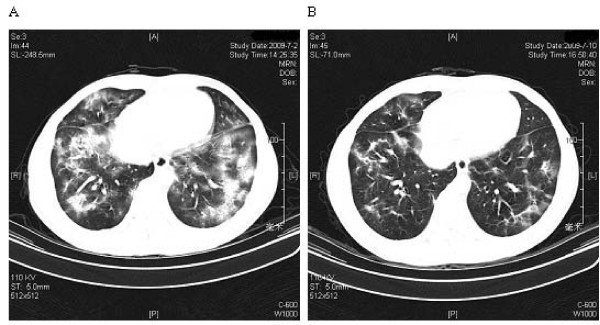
Chest CT finding of COP. A: HRCT was performed before glucocorticoids were administered, and showed patchy areas of ground-glass attenuation and consolidation in the lower lobes that contained air bronchograms. B: A follow-up HRCT was performed 8 days after glucocorticoid administration. It showed improvement in both the lungs with few residual lesions.
